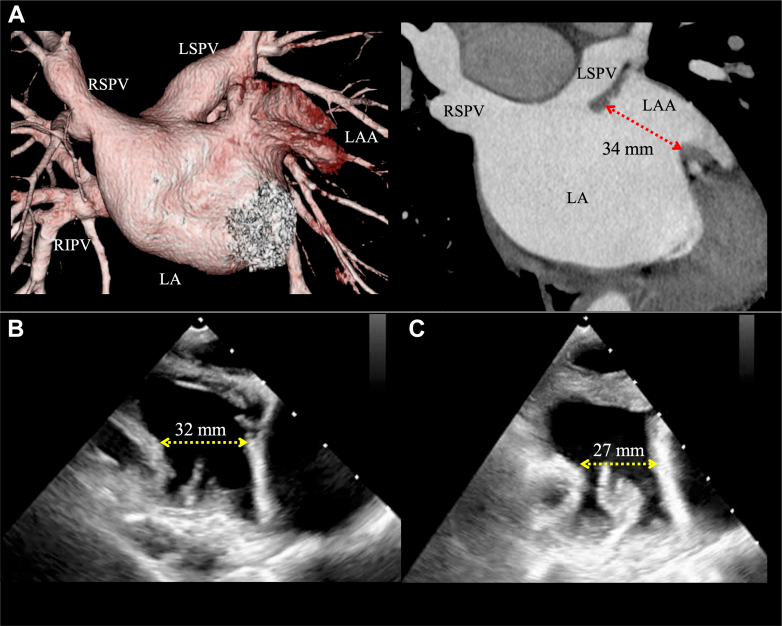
LAA Orifice Diameter Findings: Comparison Before and After PFA (A) Preprocedural cardiac CT demonstrating a cactus-shaped LAA with a maximum diameter of 34 mm. Intraprocedural TEE at a 135° angle view (B) before and (C) after PFA showed acute edema of the pectinate muscle with ostial narrowing and contour change caused by PFA, reducing the maximal diameter from 32 mm (B) to 27 mm (C). CT = computed tomography; PFA = pulsed-field ablation; TEE = transesophageal echocardiography; other abbreviations as in Figure 2.
